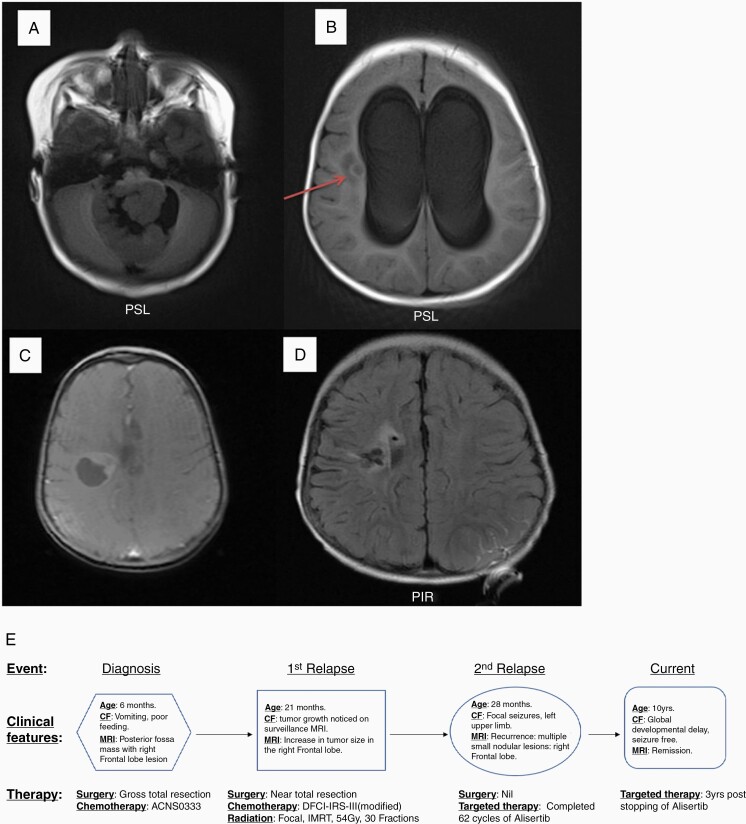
Axial T1 MRI images of the brain (A) showing posterior fossa mass measuring 4.4 cm (AP) × 2.1 cm (CC) × 4.0 cm (T) and nodule (B) measuring 0.8 cm in the right periventricular white matter with evidence of obstructive hydrocephalus. Axial T1 postcontrast MRI images at first relapse in the right frontal lobe (C) and at second relapse with increase in the size of the tumor in the right frontal lobe (D). (E) shows the graphical summary of the management of our patient from diagnosis through the two relapses.
